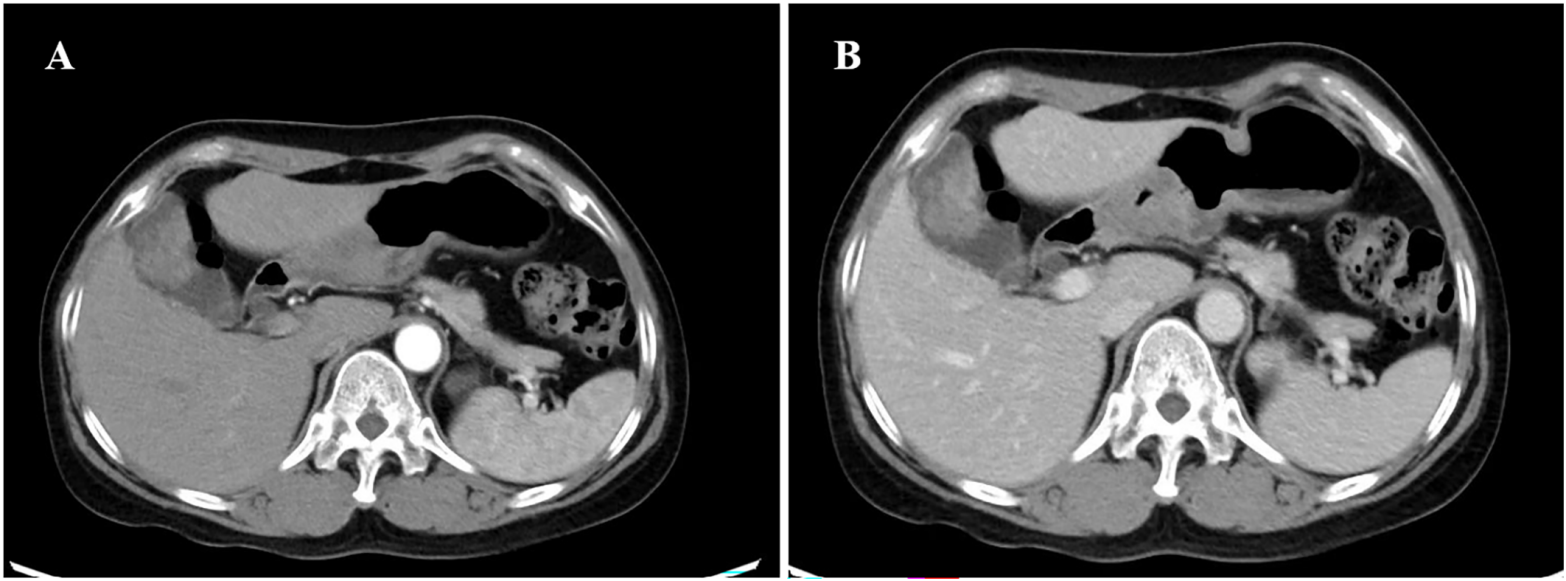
CT of the axial tomography of the abdomen and pelvis. CT scan showing a slightly high-density lesion (A, B) in the gallbladder, 3.8cm×2.6cm in size, with continuous and significant enhancement on enhanced scan. CT, computed tomography.
